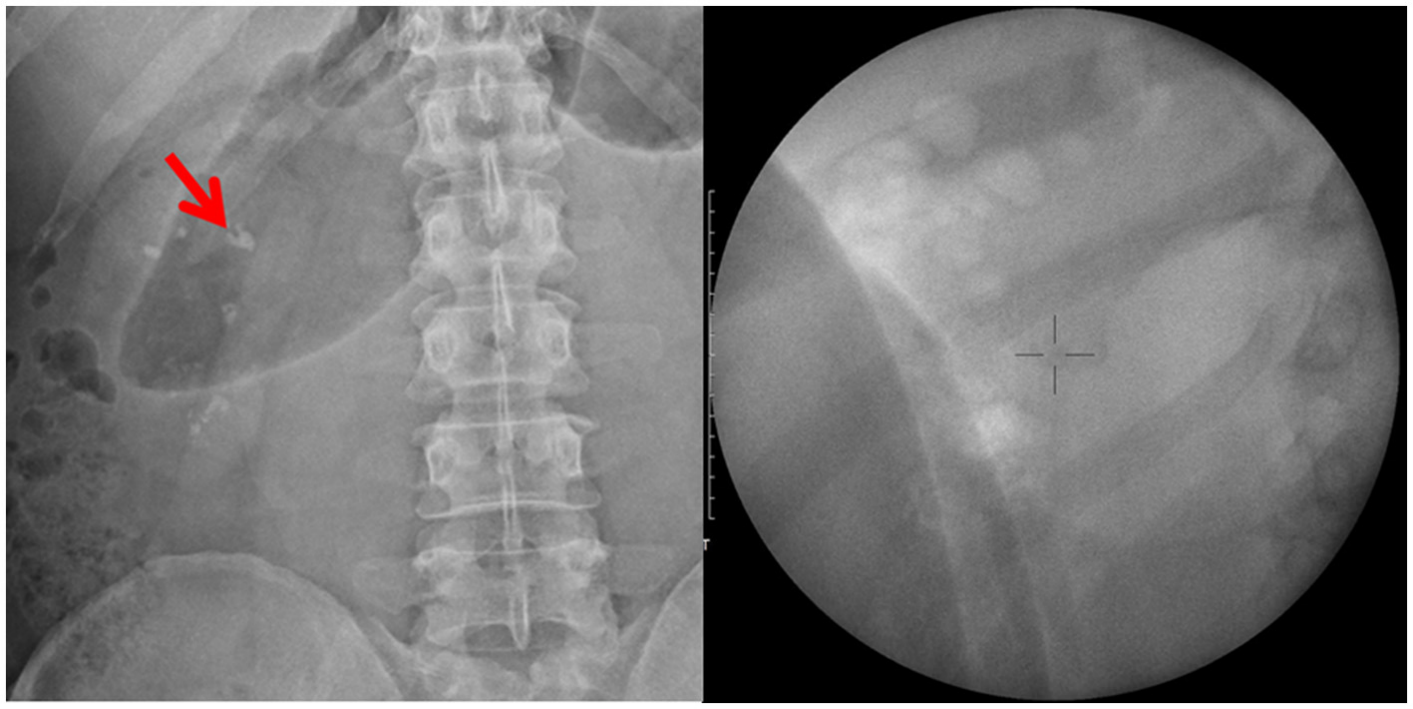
Kidney–ureter–bladder X-ray and fluoroscopy localization before shockwave lithotripsy: right renal stone with a size of 10 × 6 mm.
